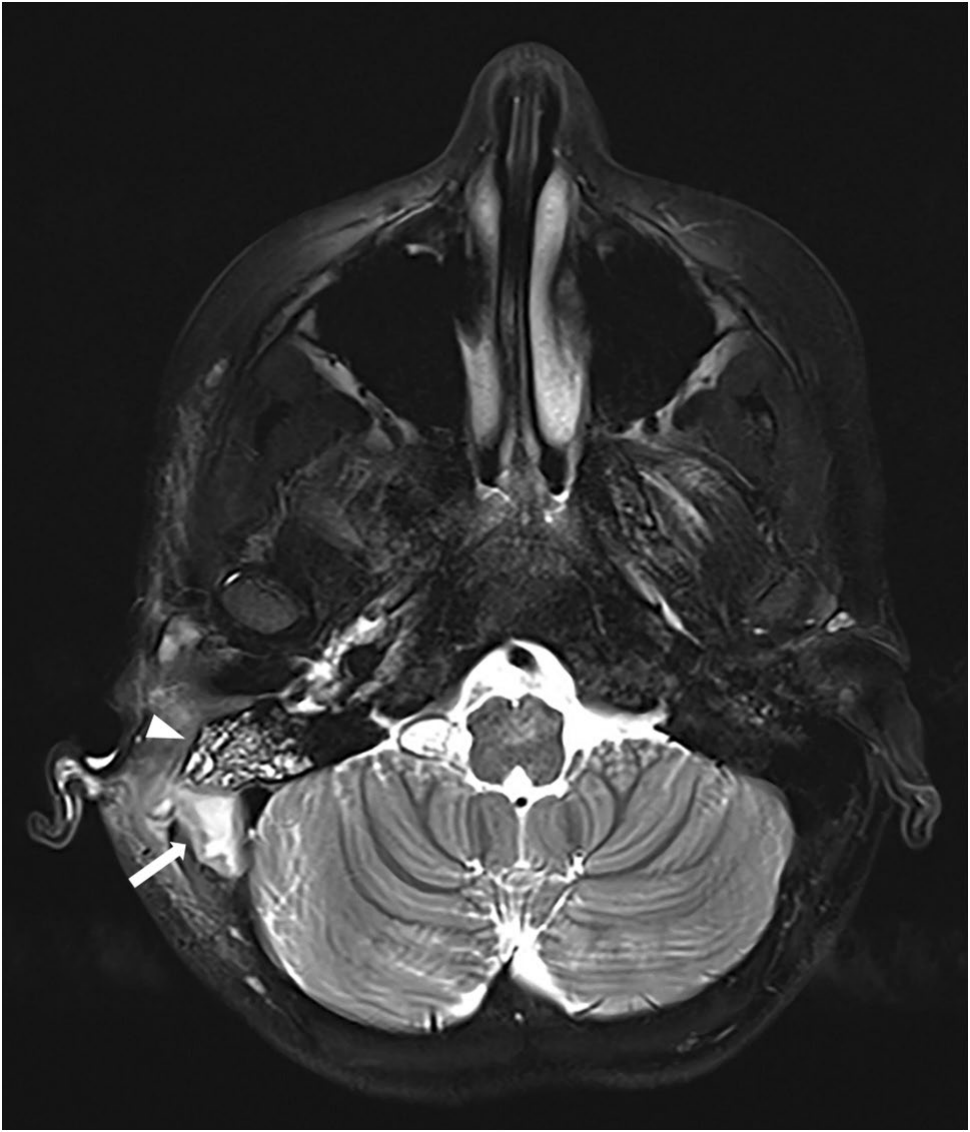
Axial T2-weighted turbo-spin-echo sequence magnetic resonance image with fat-saturation without contrast agent administration in an 8-year-old girl with mastoiditis (*arrowhead*). There is a concomitant abscess (*arrow*) on the right
